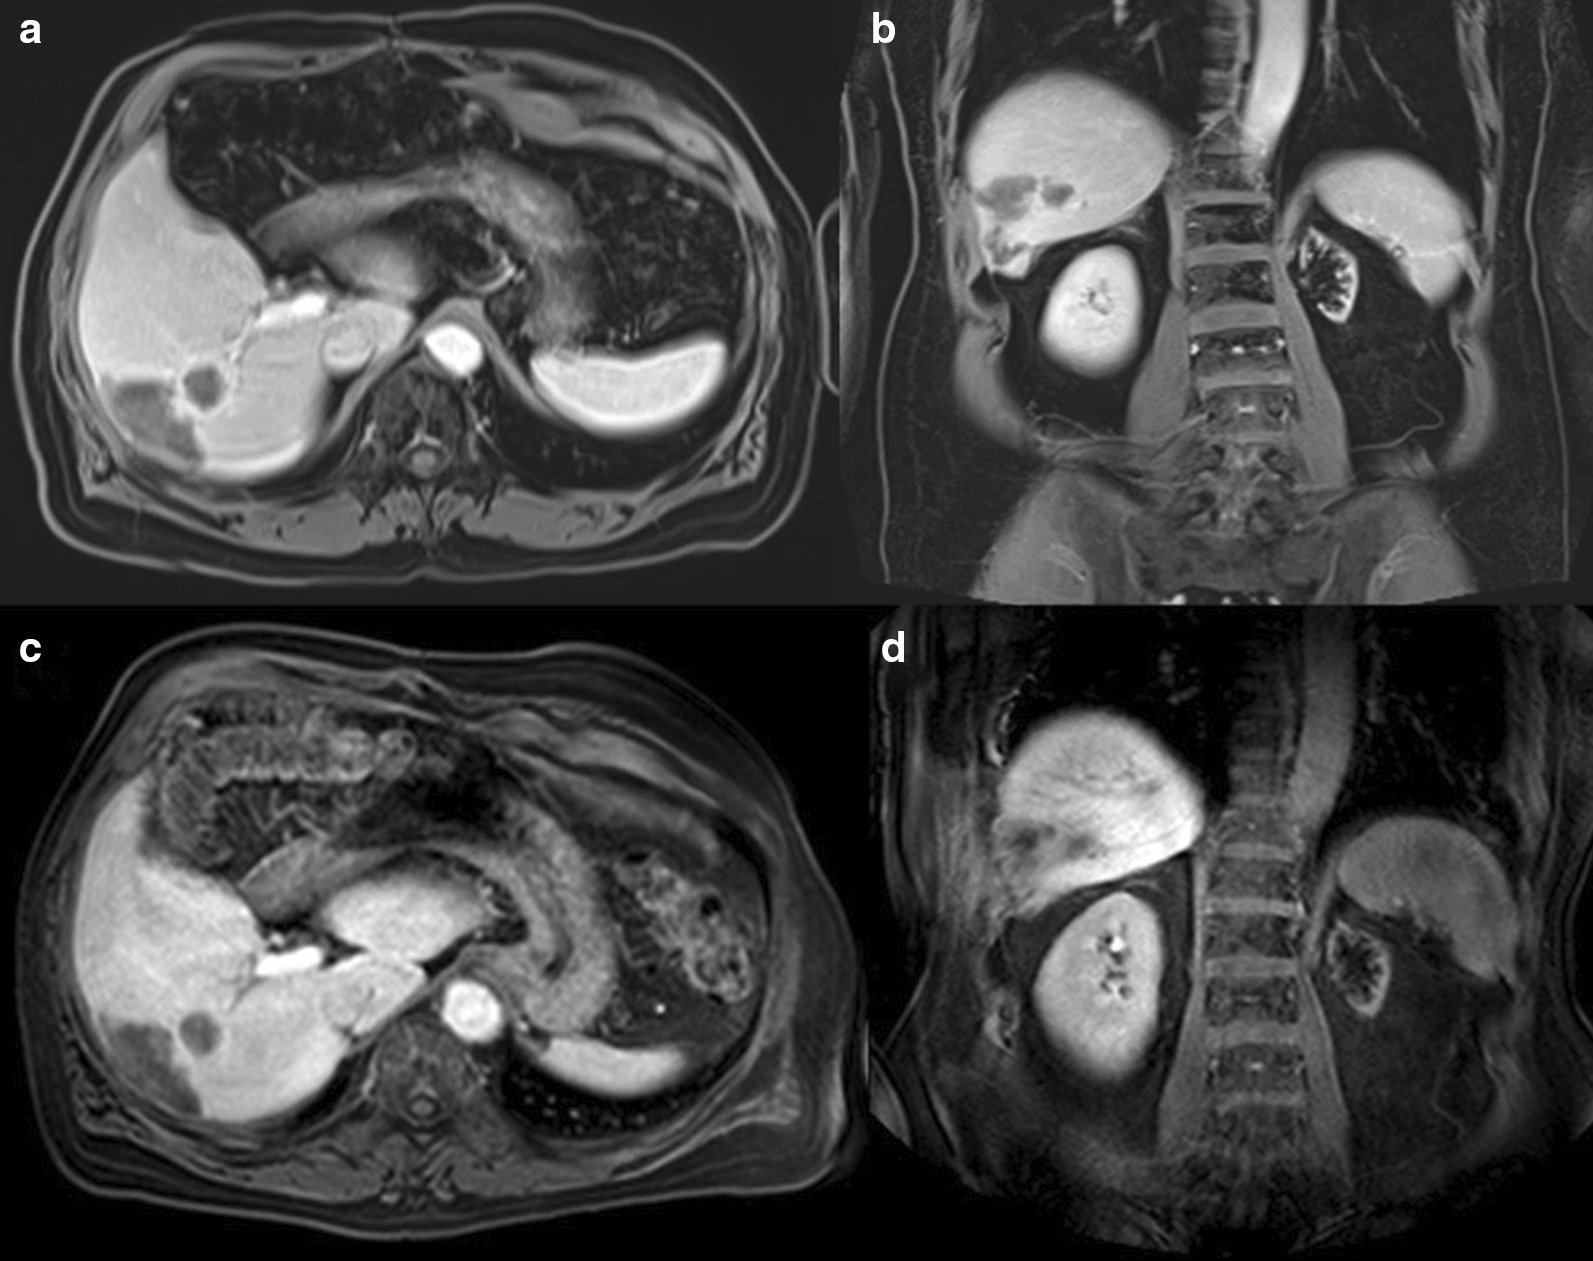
Continued post-radioembolization response. a, b Portal venous phase post-Eovist T1-weighted fat-suppressed MRI taken at 4 months and c, d 5 months post-radioembolization shows continued decrease in size of inferior right hepatic lesions
